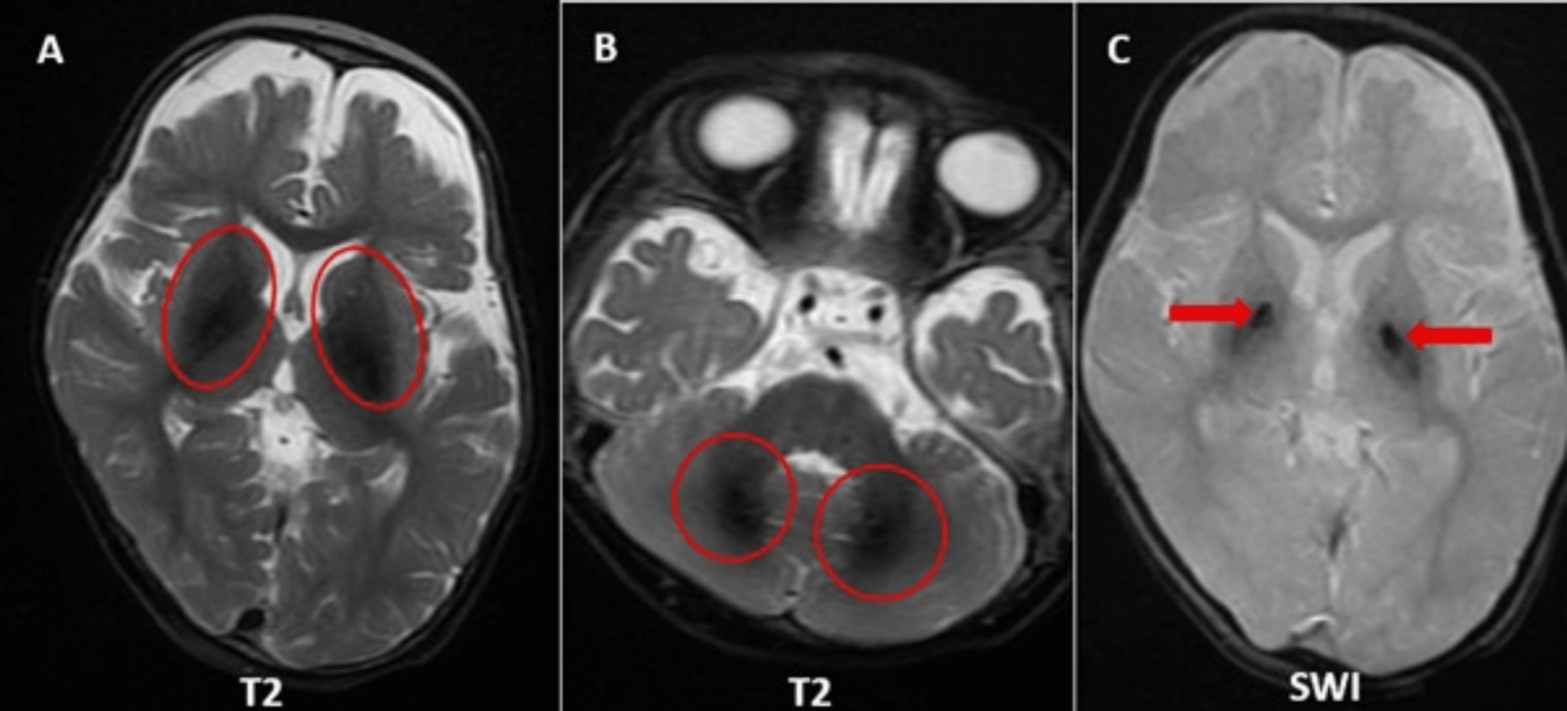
Axial sections of MRI brain (T2-weighted MRIs and SWI). (A) T2 hypointensity in the bilateral globus pallidi (red circles). (B) T2 hypointensity in the bilateral dentate nuclei (red circles). (C) Increased paramagnetic susceptibility in SWI sequences (red arrows). MRI: magnetic resonance imaging; SWI: susceptibility-weighted imaging
